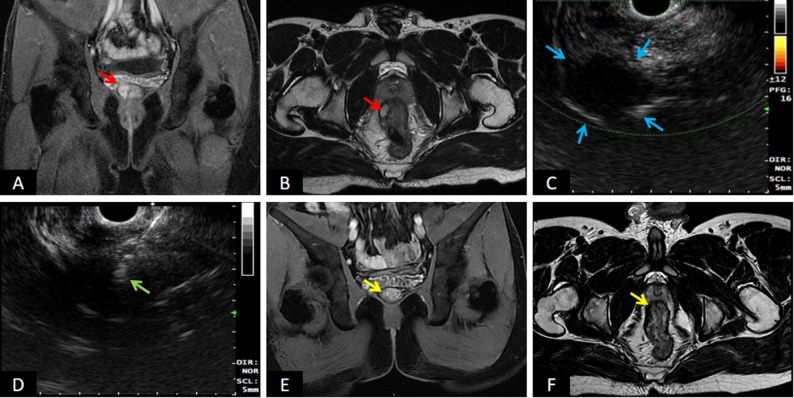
EUS-guided drainage for smaller pelvic abscesses. A Coronal pelvic MRI shows pelvic abscess (red arrow for abscess). B Transverse pelvic MRI shows pelvic abscess (red arrow for abscess). C EUS shows pelvic abscess (blue arrow for EUS view) and no blood vessels in the puncture approach. D Puncture needle is inserted into pelvic abscess under the guidance of EUS (green arrow for Puncture needle trajectory into the abscess, ). E Coronal pelvic MRI shows disappearance of abscess 18 months later (yellow arrow for post-procedure resolution). F Transverse pelvic MRI shows disappearance of abscess 18 months later (yellow arrow for post-procedure resolution)
